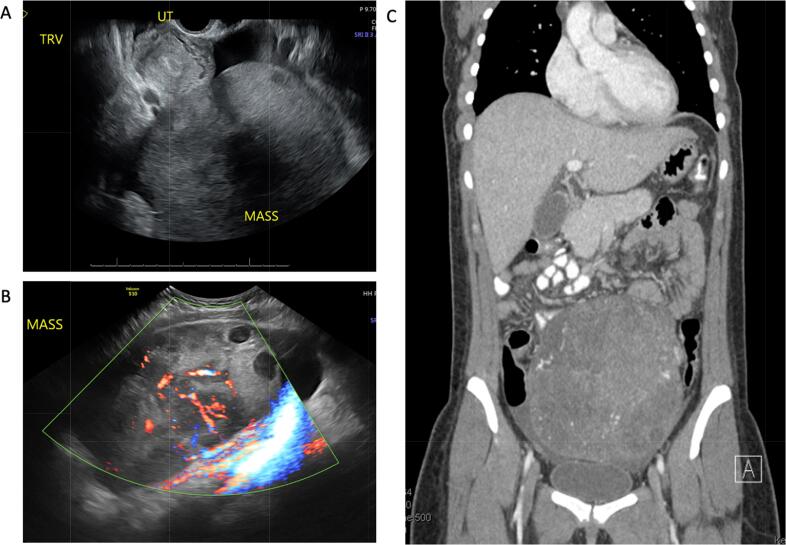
A. Transvaginal ultrasound demonstrating a large, heterogeneous vascular mass in the midline pelvis, heterogeneous echoes within the endometrium, and free fluid in the pelvis. B. Transabdominal ultrasound demonstrating heterogeneous adnexal mass with cystic areas and vascularity. C. CT of the abdomen and pelvis demonstrating 17 cm heterogeneously enhancing pelvic mass, small volume ascites.
